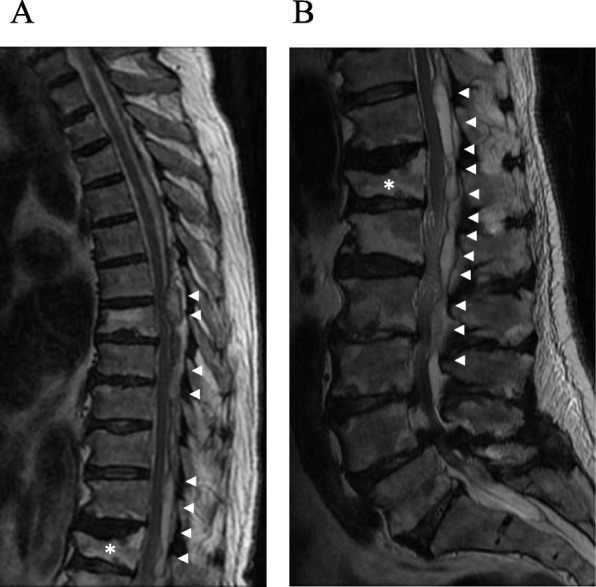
a, b Magnetic resonance imaging (T2) performed on admission. Fluid retention is observed in the epidural space behind the Th6-L3 spinal canal (arrow). Compression of the spinal cord near Th6/7, Th11/12, and L2/3 due to fluid retention is shown. Vertebral compression fractures can also be seen at Th12 (asterisk)
